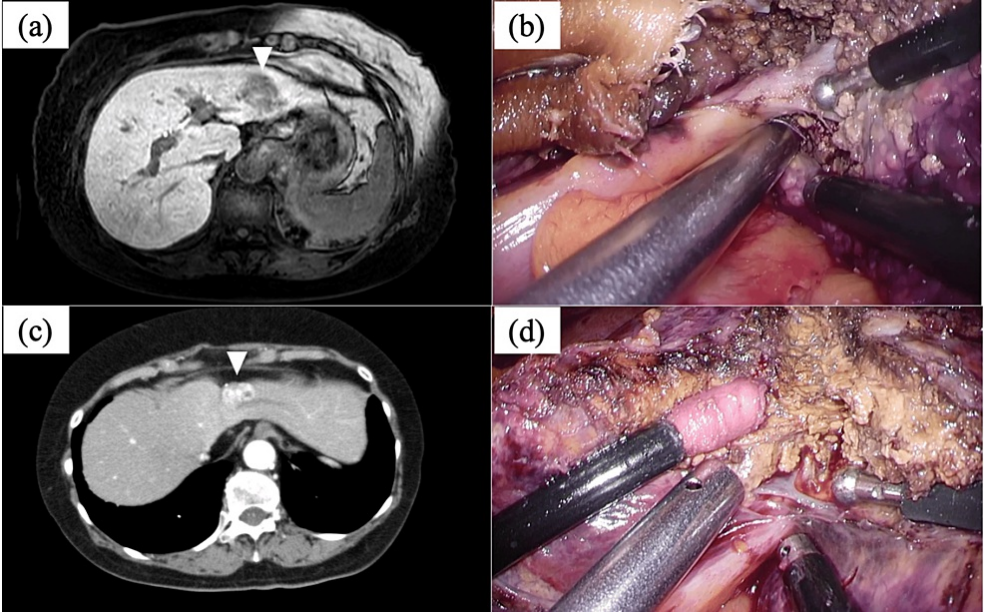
MRI image and intraoperative findings of cases 3 and 6 (a, b) In case 3, a 3-cm-sized tumor in the lateral section of the liver was detected in contrast-enhanced MRI (arrowhead), and left lateral sectionectomy utilizing WJS was performed. (c, d) In case 6, a 2.5-cm-sized tumor was detected in S2 and the patient underwent left lateral sectionectomy using WJS. MRI; magnetic resonance imaging, WJS; water jet scalpel, S2; segment 2
